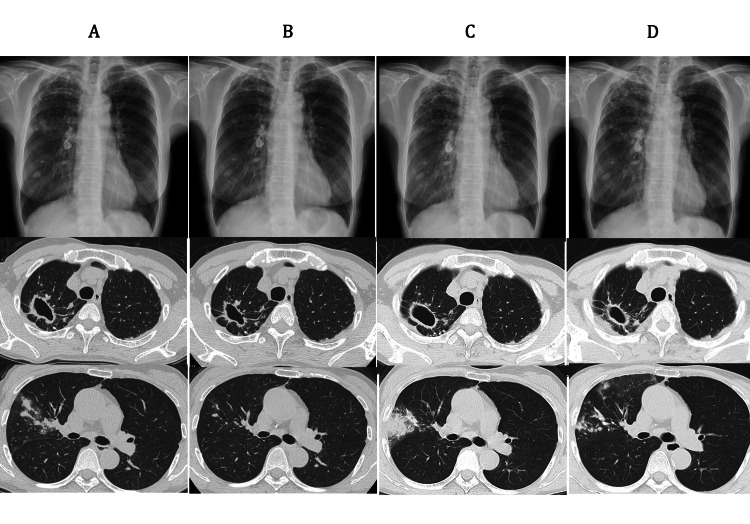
Chest roentgenogram and chest computed tomography (CT) (A) Disease onset: chest CT showed a cavity with a thickening of the wall in the right upper lobe, continuous infiltration from the right upper lobe to the middle lobe, and a nodule in the right lower lobe. (B) After the first treatment: a marked reduction in infiltration into the right lung was seen. The nodule in the right lower lobe remained unchanged. (C) At the time of recurrence: thickening of the cavity wall was seen, with increased infiltration into the right lung. (D) After 19 months of treatment: the infiltration had mostly resolved, but the shadows were more evident than after the first treatment. The nodule in the right lower lobe remained unchanged. This nodule was considered an old shadow.
